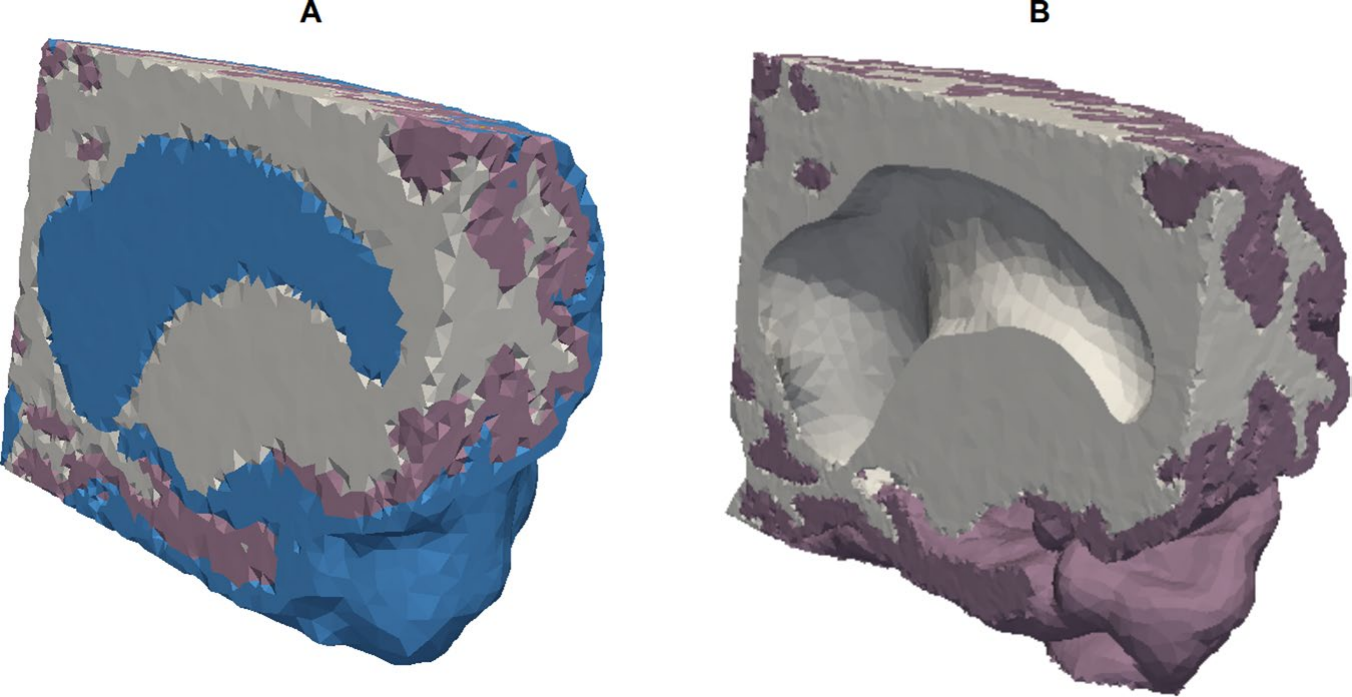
The leftmost image (A) shows the mesh created from the baseline MRI image with three domains, while the rightmost image (B) shows the mesh created from the baseline MRI image with two domains. The blue domain corresponds to CSF domain, Ω_*CSF*_, the purple domain corresponds to grey matter, Ω_*GM*_, and the white domain corresponds to white matter, Ω_*WM*_. Both images concern NPH1.
